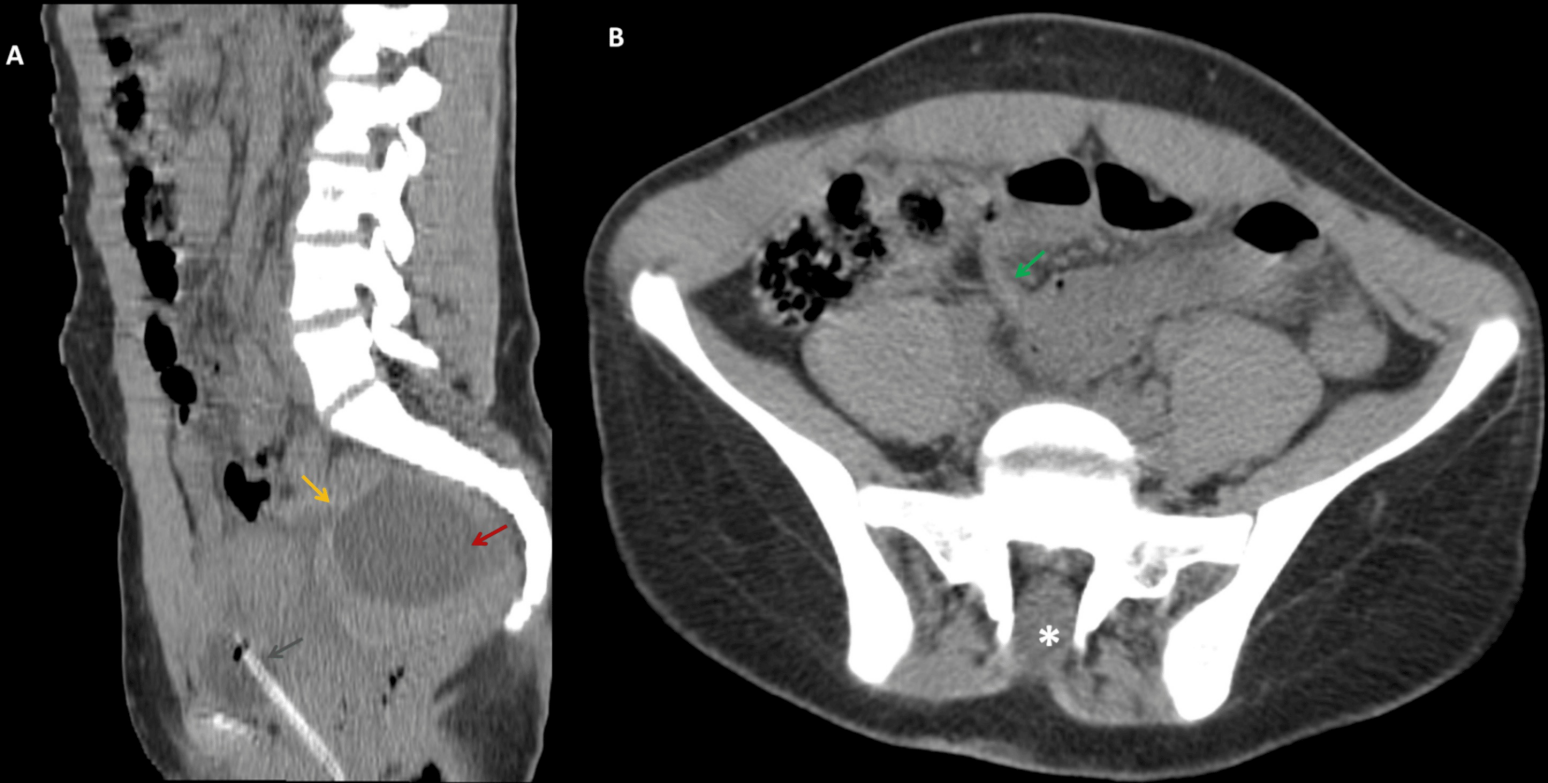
Non-contrast CT imaging of a pelvic mass with a normal appendix A. Sagittal view of a non-contrast CT scan, showing the previously described pelvic mass (orange arrow) with a central cystic lesion within it (red arrow). Note the urinary bladder catheter in position (blue arrow). B. Axial abdominal CT slice demonstrating a normally shaped appendix (green arrow). Additionally, note the posterior spinal defect (white asterisk).
